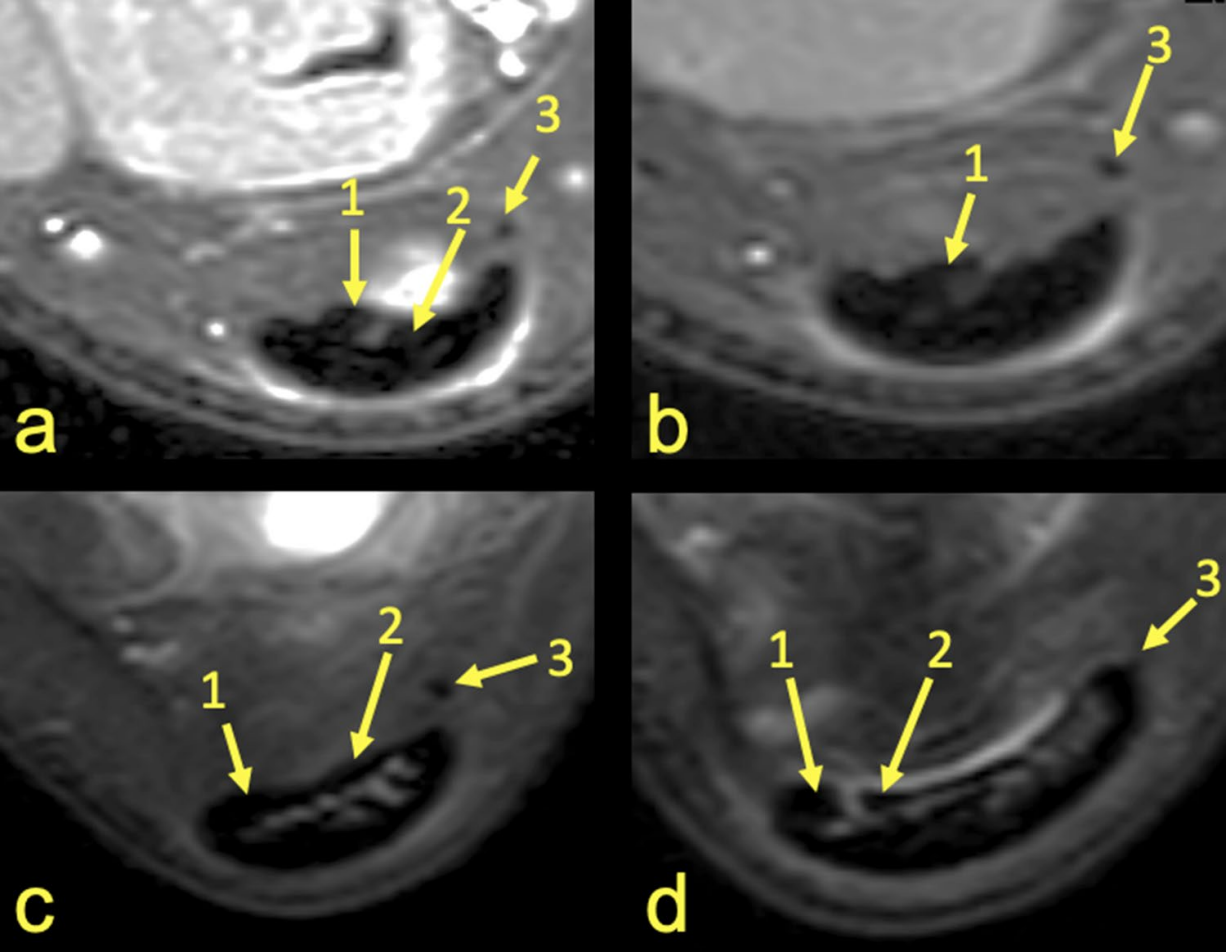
MRI right ankle, PD FS at the level of the talus (a–c), (a)—the highest section, (d)—the lowest section and at the level of the deep calcaneal bursa just above the calcaneus (d). A 17-year-old female 1 week after ankle sprain, pain posteriorly to the lateral malleolus. 1—S-Sol, 2—S-LGC, 3—the plantaris tendon (type 4).
